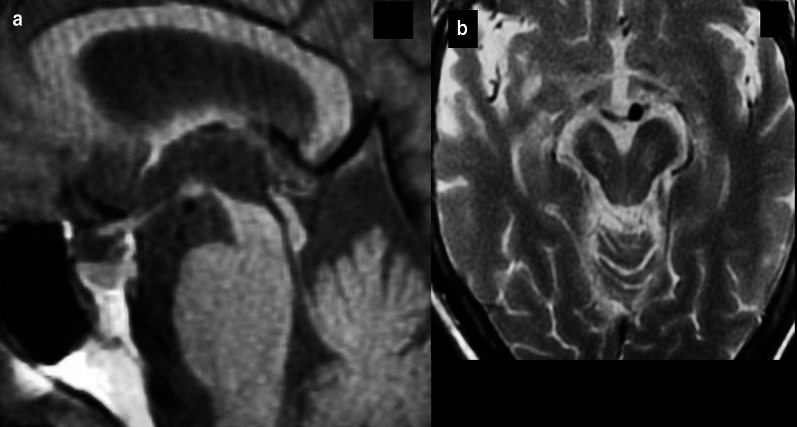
Sagittal T1-weighted image in the midline coned to the brainstem demonstrates midbrain atrophy creating the so-called hummingbird sign of PSP (a). Axial T1-weighted image at the level of the midbrain demonstrates decrease in the AP dimension of the midbrain and widening of the interpeduncular cistern; the so-called morning glory sign (b)
